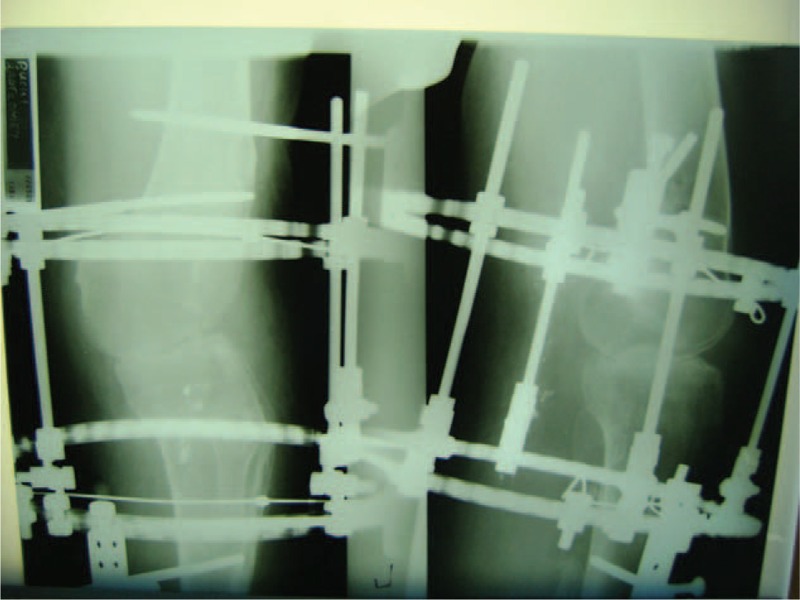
The x-ray of the left knee 2 days after the surgery.
